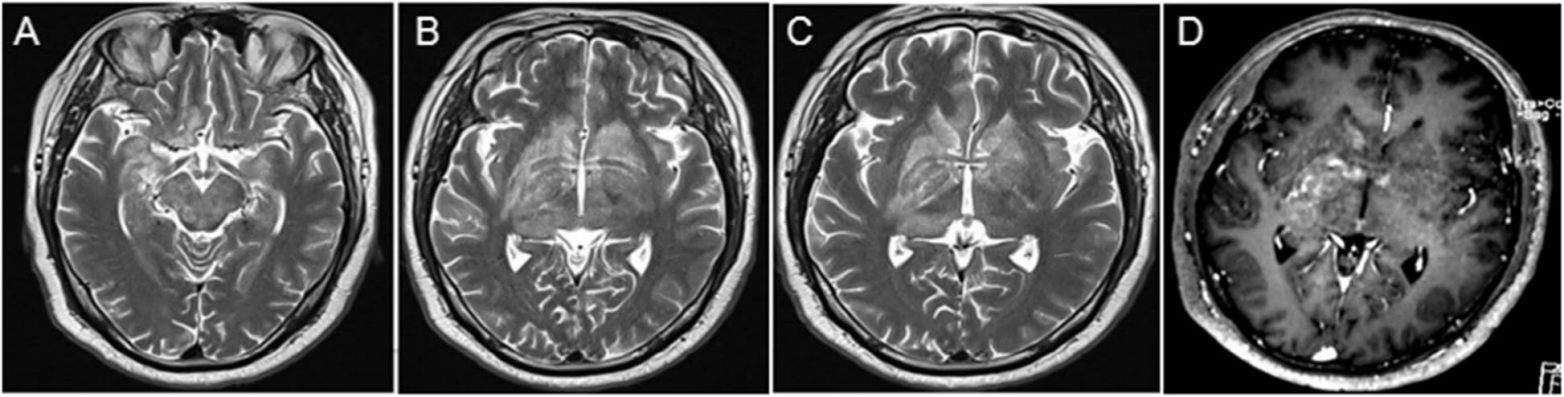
MRI at the first admission. a-c T2-weighted sequence, diffuse hyperintense infiltrating lesion, involving the bilateral basal ganglia, thalamus, brainstem, hippocampus, temporal lobe, and left insula. d T1 weighted image after contrast: demonstrating heterogeneous enhancement
